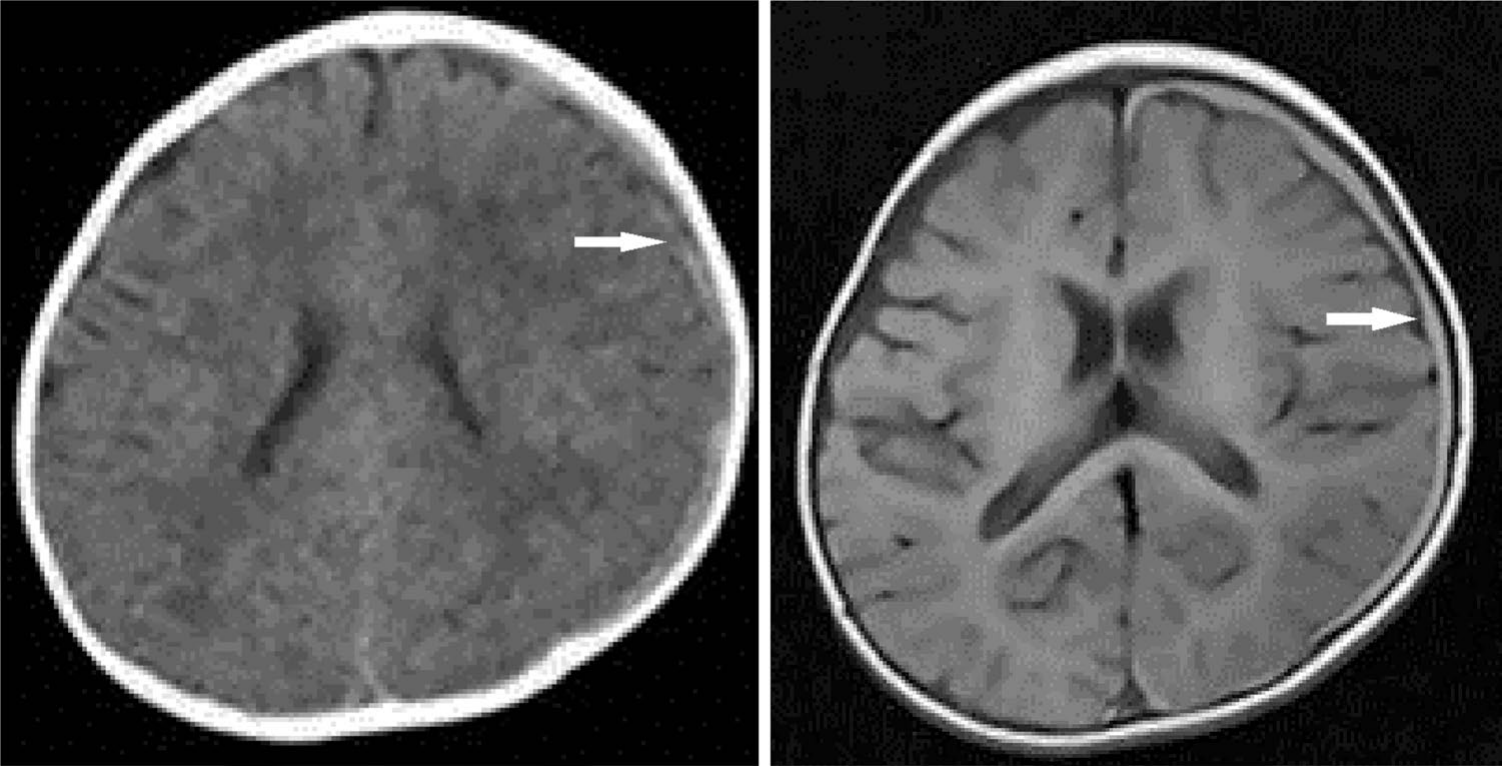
Case 1, male aged 8 months. Left: CT on arrival showing thin, irregularly shaped SDH, indicating an unclotted SDH on the left side (arrow). Right: MRI (FLAIR) 12 days after symptom onset demonstrating a thin, film-like, high-intensity SDH on the left convexity (arrow). No mass effect or parenchymal abnormality was observed on the other sequences (not shown)
